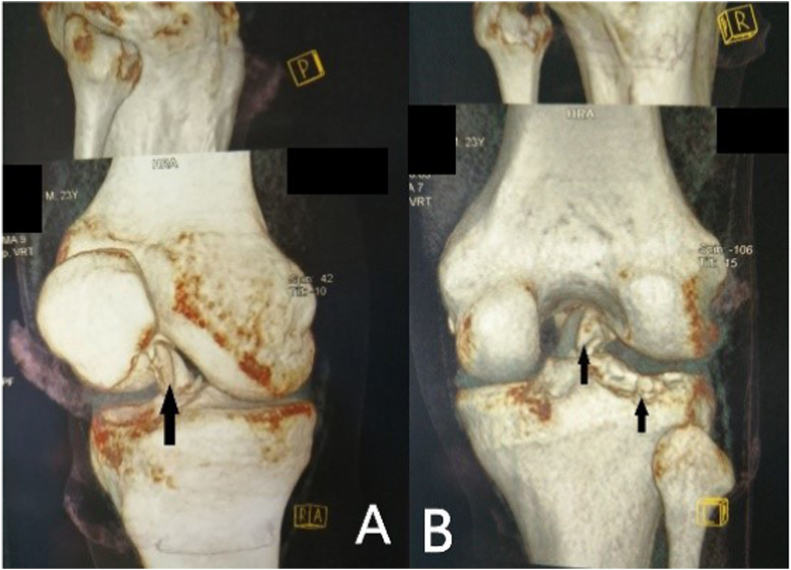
CT with a reconstruction of the right knee; anterior (A) and posterior (B) views reveal irregularities in the posterio-latero-central segment of the tibial plateau accompanied by an avulsion fracture displaces and inserts into the anterior compartment of the knee (black arrows).
